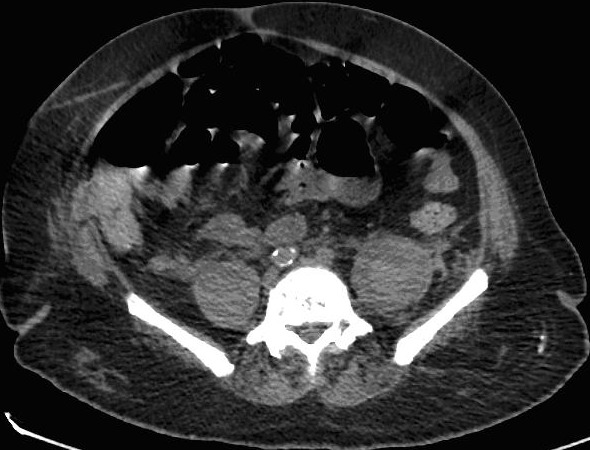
Nonenhanced CT scan examination showed heterogeneous organized collections identified along the right and left psoas muscles causing focal contour bulge representing haematoma, minimal fat stranding surrounding the psoas muscles, more significant on the left side
